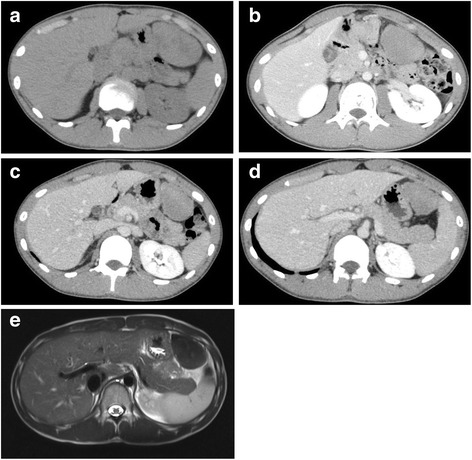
Computed tomography (CT) examinations revealed a solid mass that gradually shrank. This mass measured approximately 74 mm × 45 mm in September 2014 (a), 57 mm × 44 mm in December 2014 (b), 56 mm × 37 mm in March 2015 (c), and 49 mm × 34 mm in June 2015 (d). MRI examination revealed isointensity to the spleen on T1-weighted images and slightly inhomogeneous hypointensity on T2-weighted images (e)
